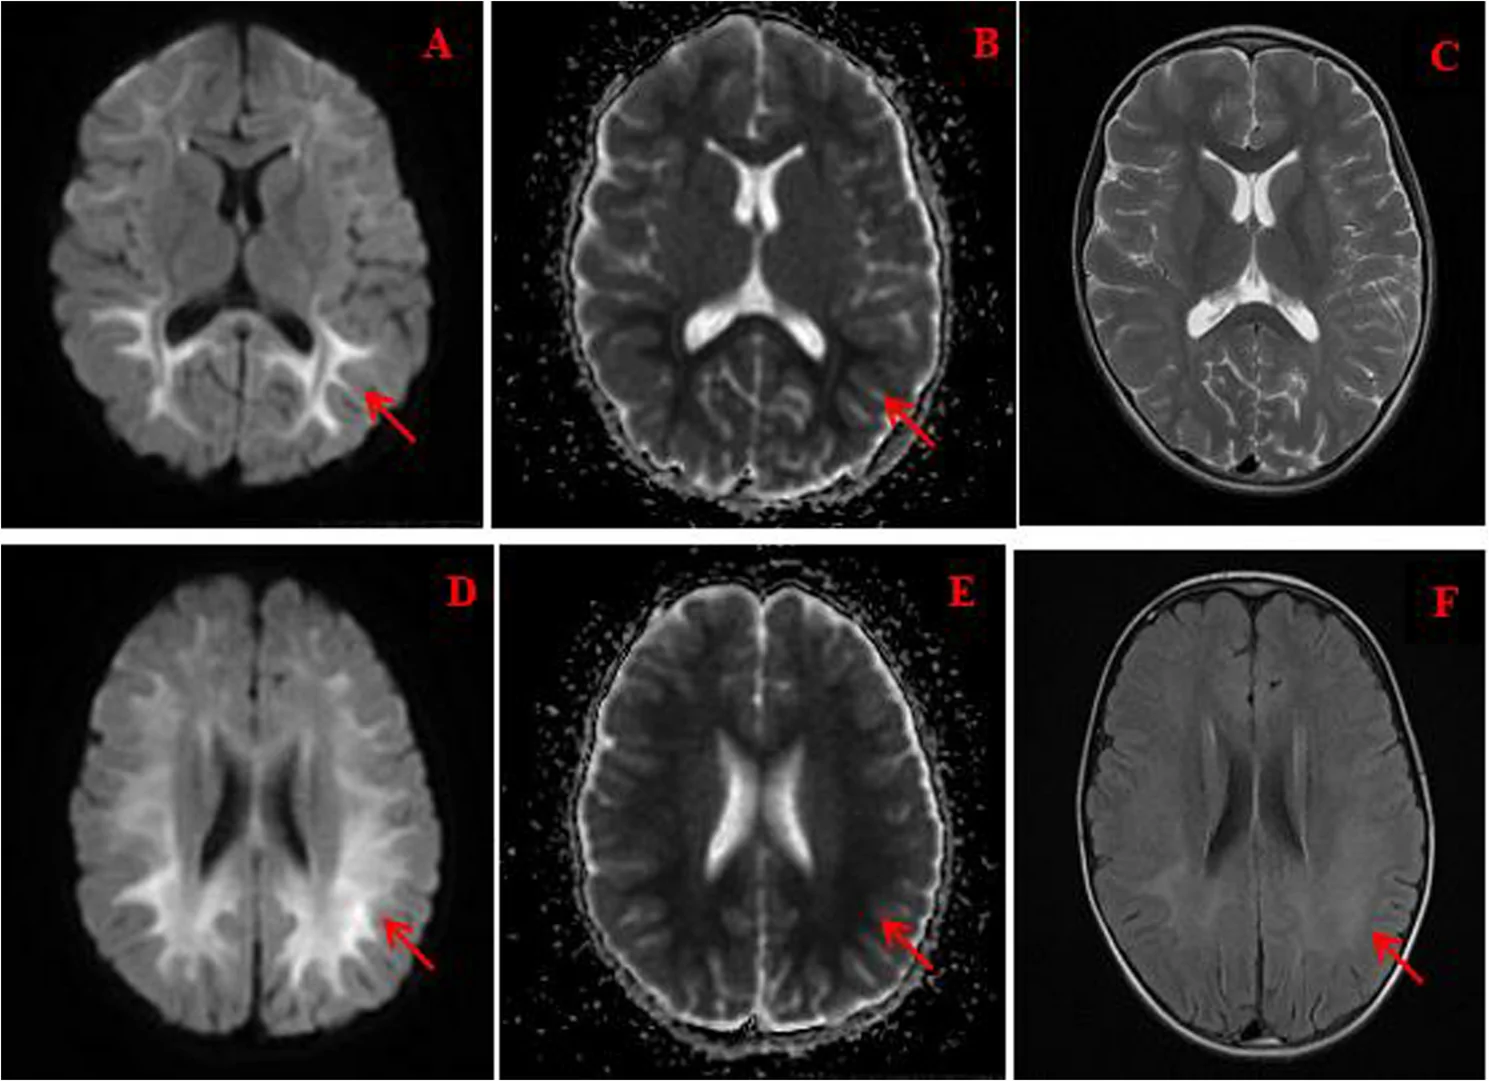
Brain MRI results of children with hypersarcosinemia (March 12, 2025, one and a half months after discharge). A and D: Large patches of DWI hyperintense signal in the subcortical white matter of the bilateral cerebral hemispheres (arrows). B and E: Large patches of subcortical white matter in the bilateral cerebral hemispheres showed hypointense on ADC maps (arrows). C: T2-weighted images showed no abnormalities. F: Slight FLAIR hyperintensity in the white matter of the posterior horn of the bilateral ventricles (arrows). MRI, magnetic resonance imaging. DWI, diffusion-weighted imaging. ADC, apparent diffusion coefficient. FLAIR, fluid-attenuated inversion recovery
